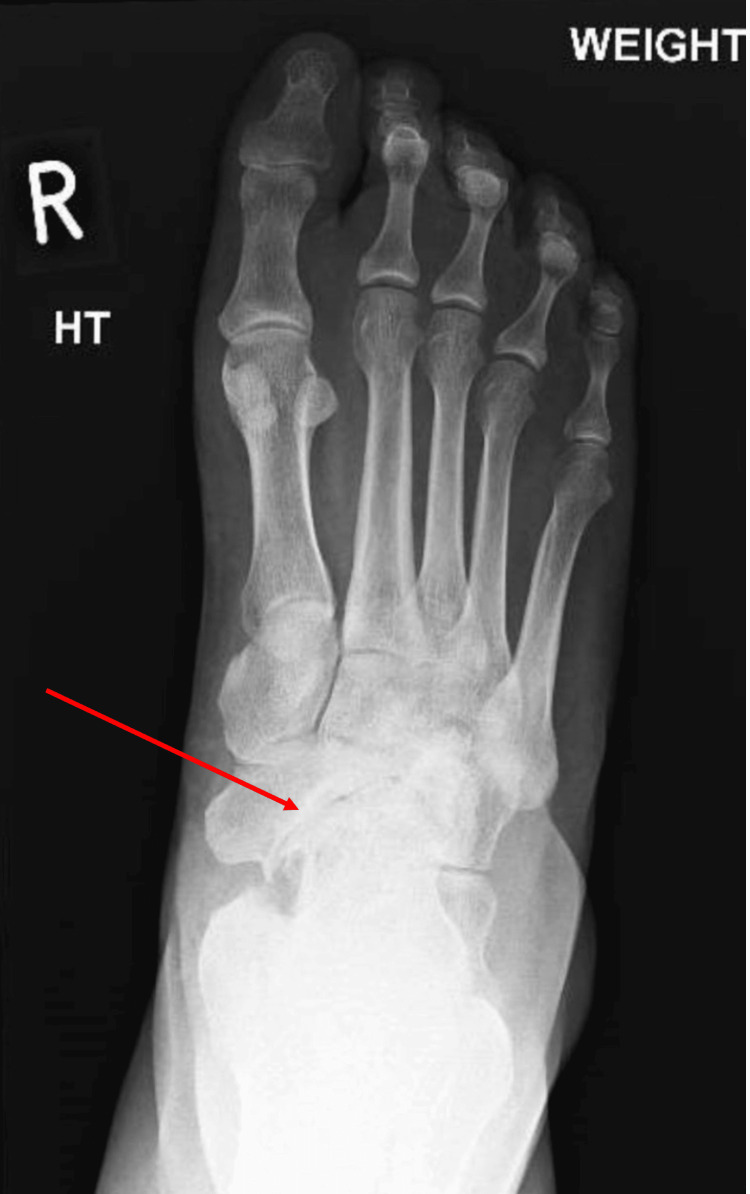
Antero-posterior radiograph of the right foot, arrow demonstrates secondary arthritic changes
